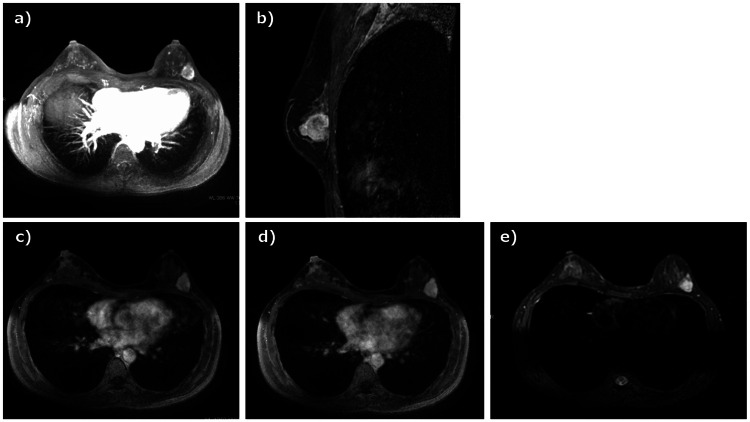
MRI findings in this case. MRI shows a 31mm well-defined oval mass that exhibits contrast enhancement with a heterogeneous fast-persistent pattern in the upper outer area of the left breast. (a) Maximum intensity projection image. (b) Sagittal section image. (c) Initial phase of dynamic contrast-enhanced MRI. (d) Delayed phase of dynamic contrast-enhanced MRI. (e) Fat-suppressed T2-weighted image.
